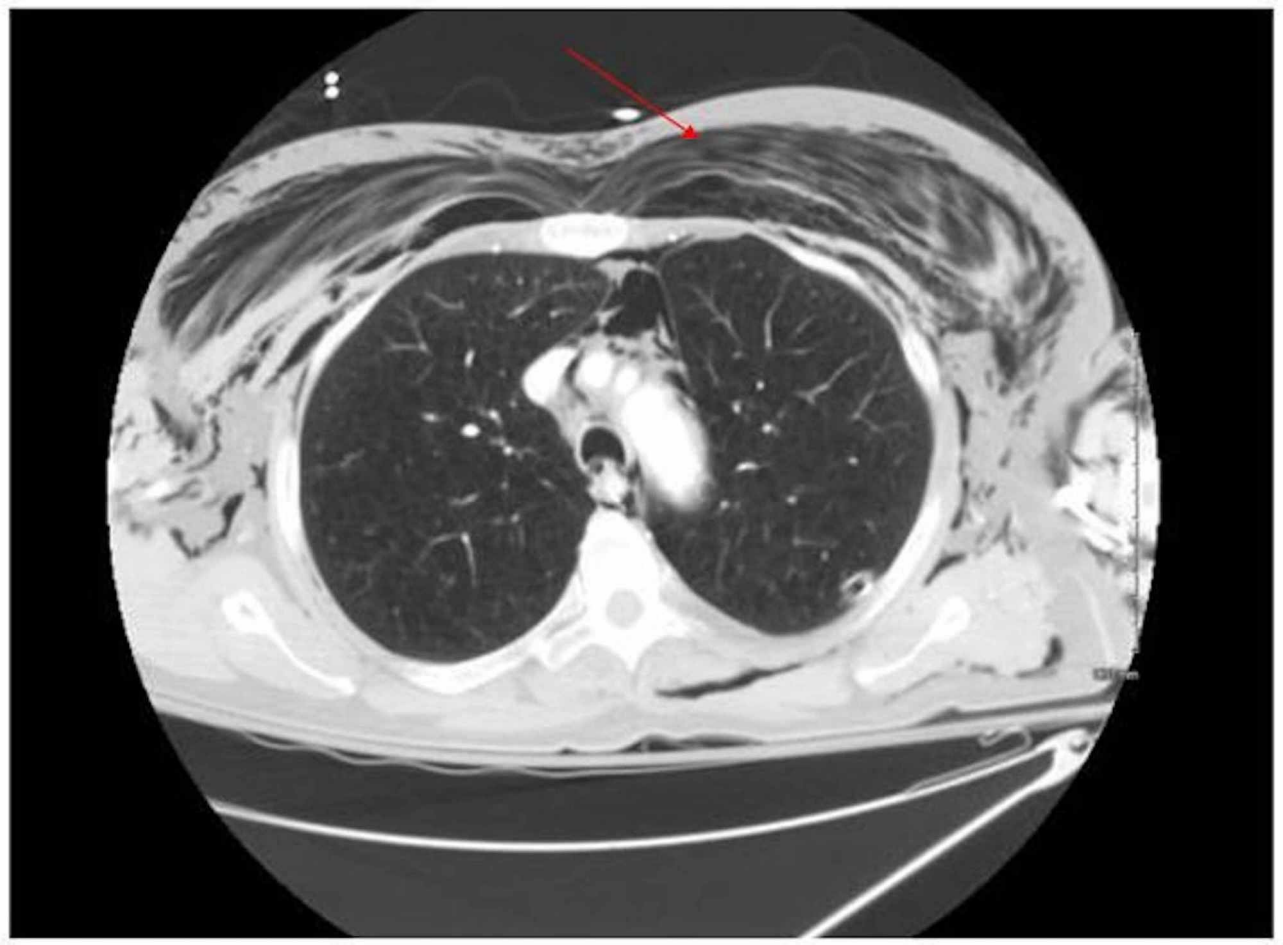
Repeat CT scan of the patient showing extensive subcutaneous emphysema Repeat CT on day 2 after biopsy and day 4 after failed biopsy, showing extensive subcutaneous emphysema (arrow) and pneumomediastinum, with the chest tube located in the posterior left lung.
